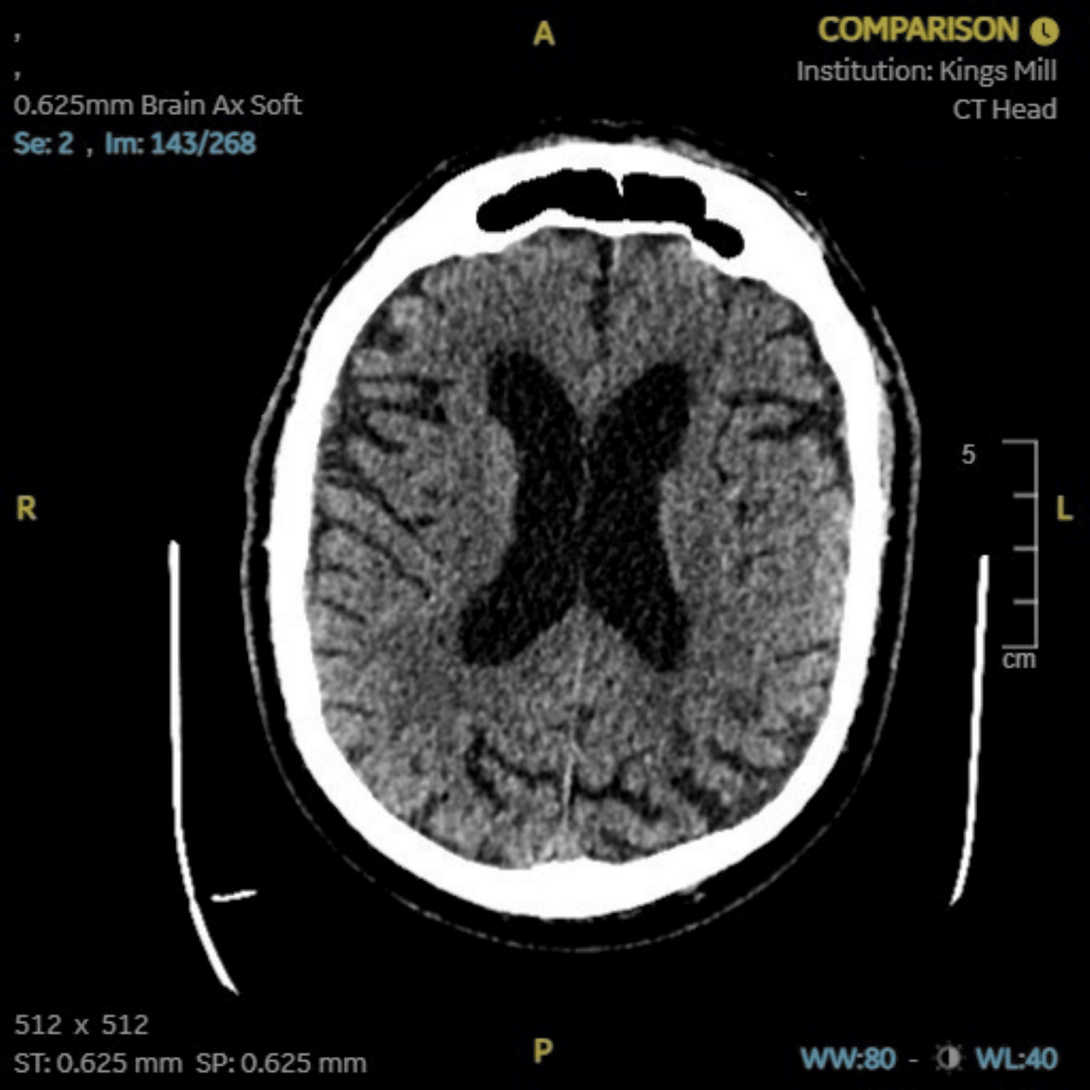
CT scan of the head showing no acute intracranial pathology
